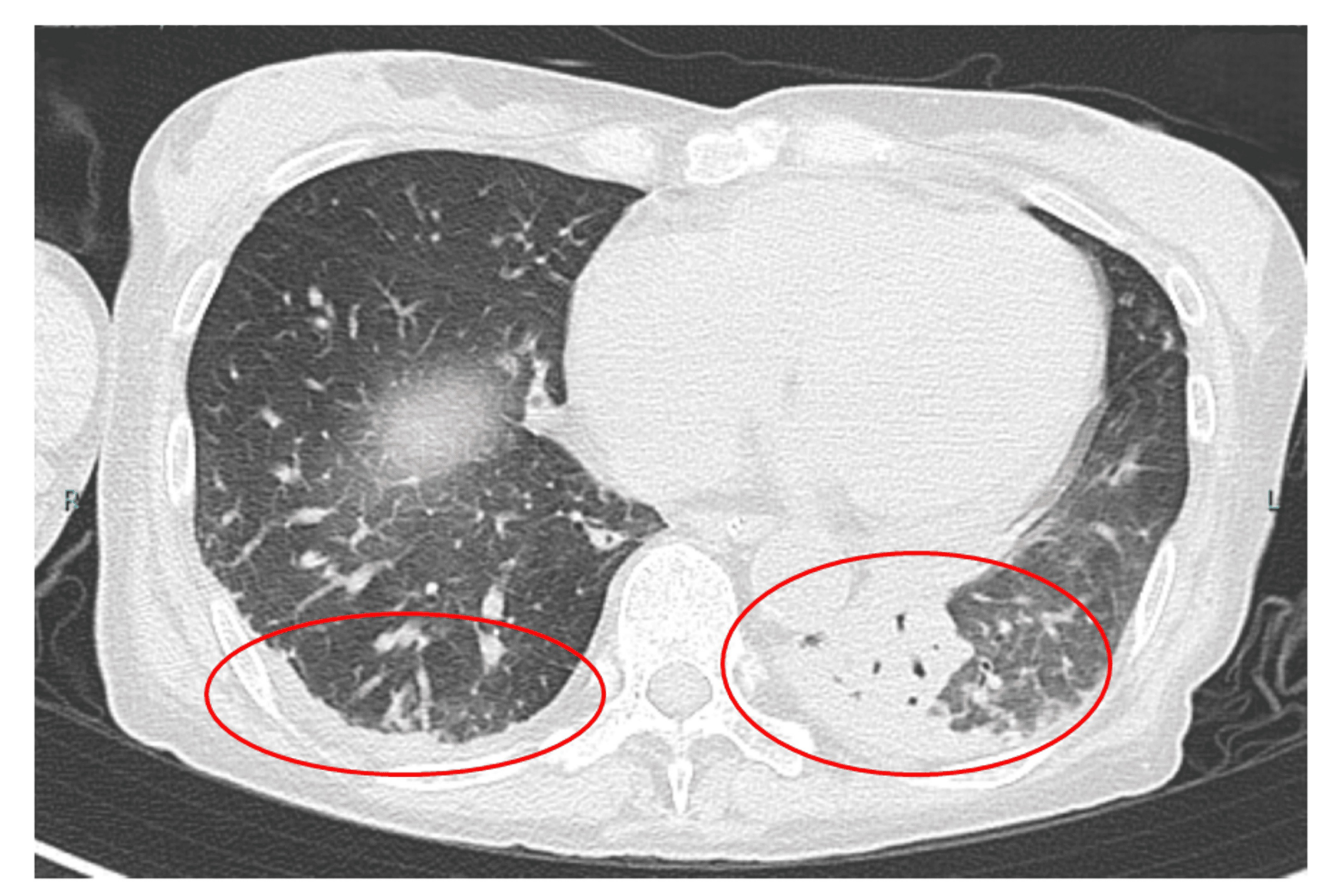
Coronal section of a chest CT showing bilateral lower lung wetting and increased bronchial translucency in the left lower lung.
